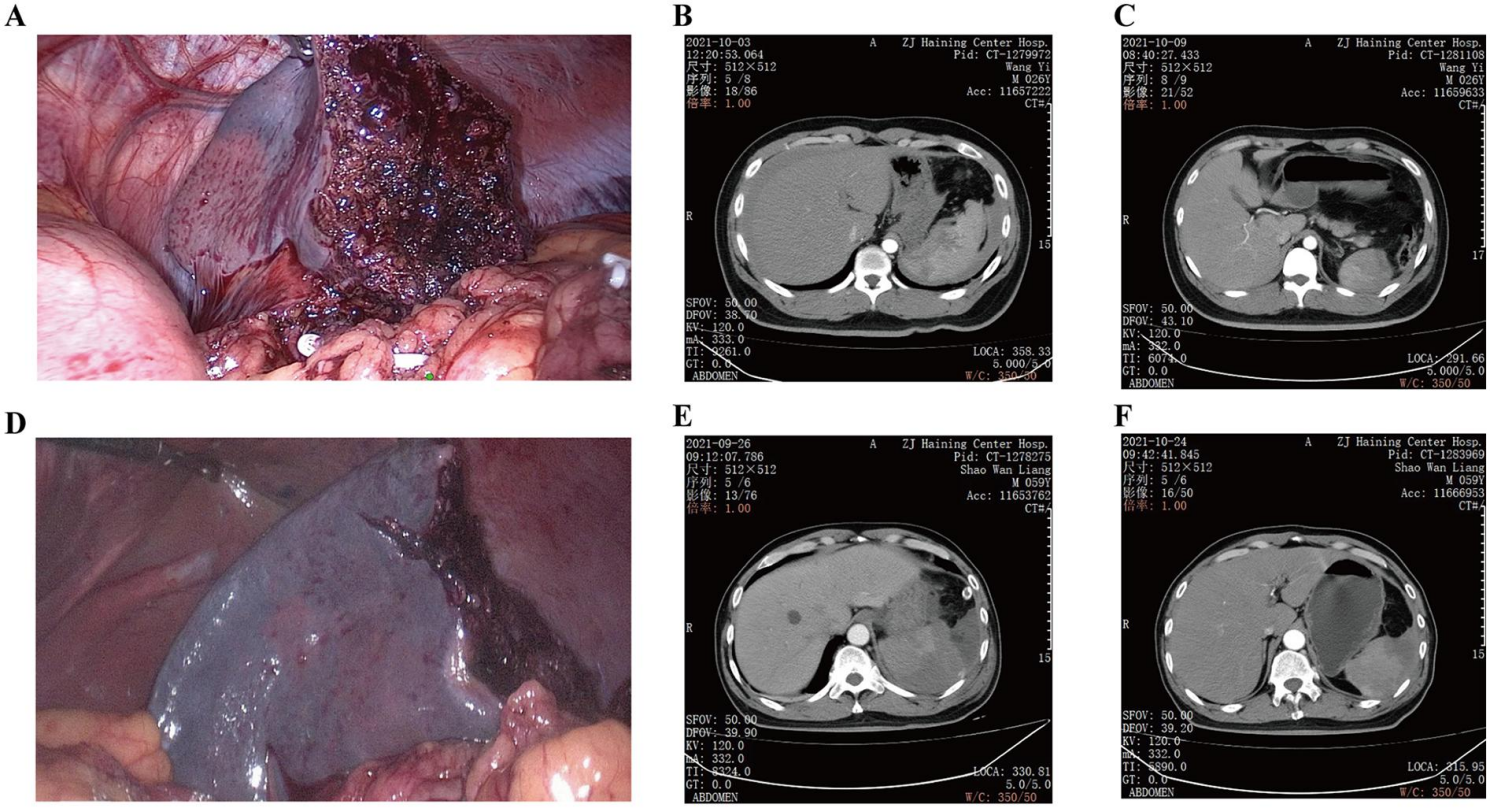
Cases of partial splenectomy with preserved omental blood supply. (A) Post-excision trauma with slight ischemic manifestation of the stump; (B) pre-operative enhanced CT suggesting injury of the splenic hilum; (C) post-operative CT on the 6th day, suggesting a good blood supply of the residual spleen; (D) partial resection of the residual spleen with dark blood color and ischemic manifestation; (E) re-examination of CT on the 7th day after the operation suggesting a partial ischemic manifestation of the residual spleen; (F) re-examination of the CT on the 30^th^ day after the operation. CT, suggesting improvement of ischemia and partial liquefaction of the residual spleen.
